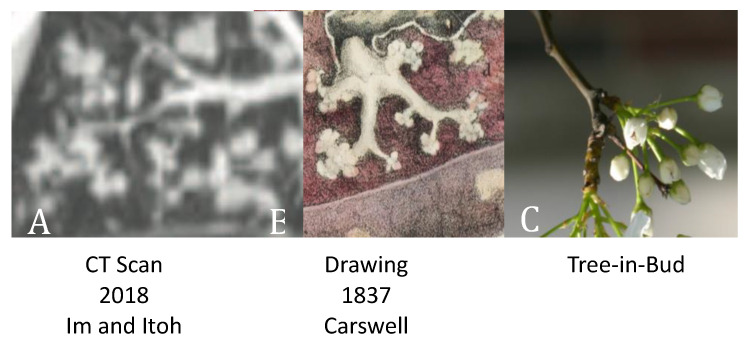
Tree-in-bud sign on CT scans was recently rediscovered as the characteristic early lesion of PPTB. It was described by Carswell in 1837 and by X-rays in the 1920s [42,46,50]. Today, it is recognized as characteristic of advancing post-primary TB.
